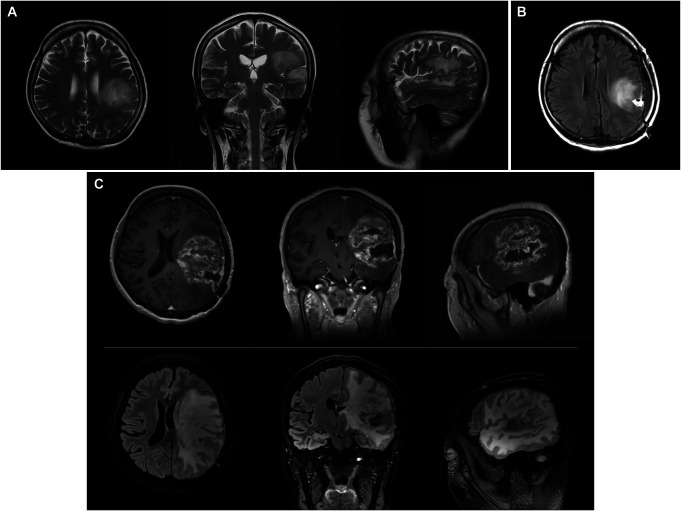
A, Axial, coronal, and sagittal fluid-attenuated inversion recovery images taken before the first surgery at a former medical facility. B, Axial fluid-attenuated inversion recovery image taken after the first surgery at a former medical facility. C, Axial, coronal, and sagittal gadolinium-enhanced T1-weighted (top) and fluid-attenuated inversion recovery (bottom) images taken before the second surgery.
